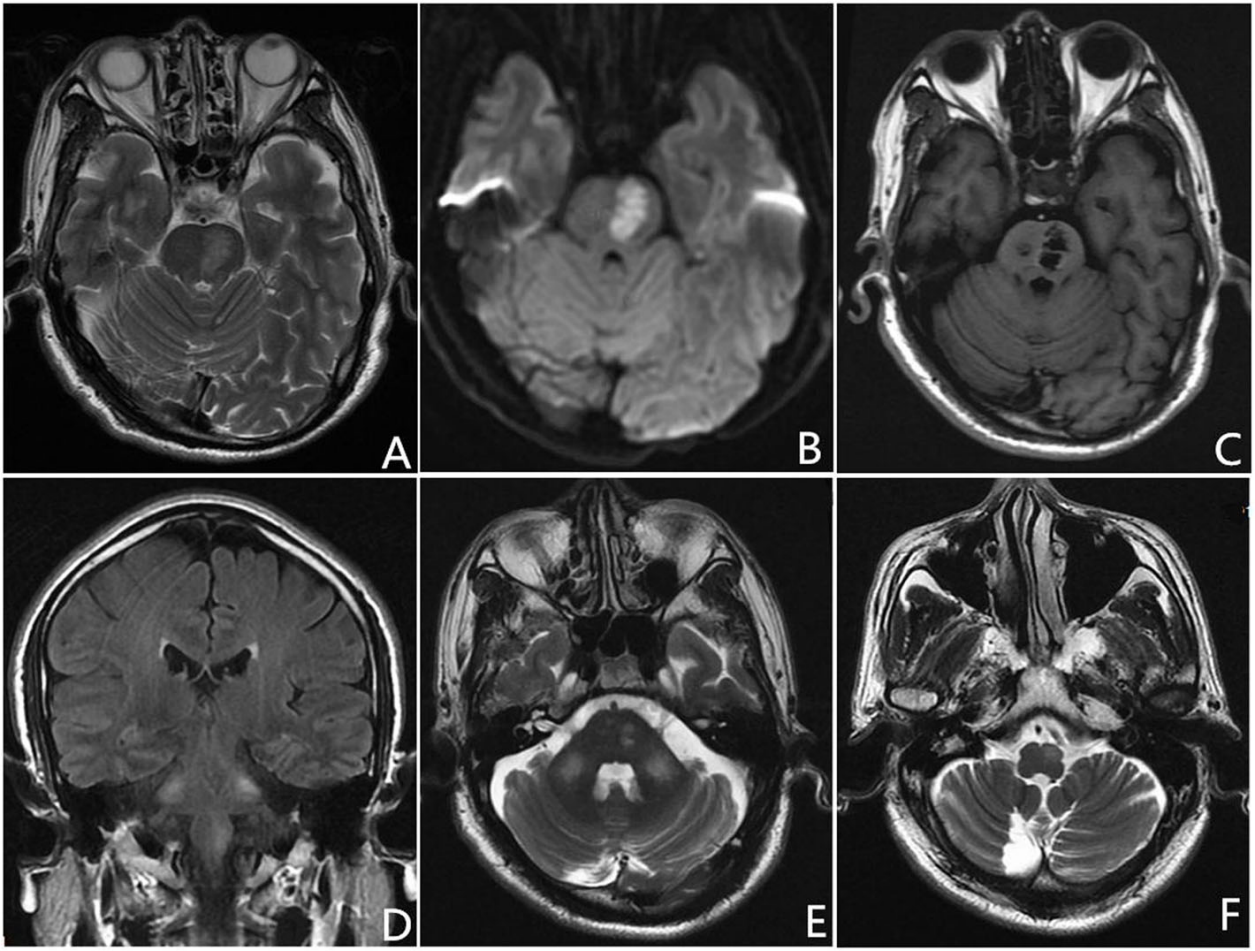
Axial T2-weighted and DWI images show acute pontine infarction (a, b). On 3-month follow-up MRI, axial T1-weighted image demonstrates an old paramedine pontine infarction (c). Coronal fluid-attenuated inversion recovery and axial T2-weighted images reveal hyperintensiy in the left inferior olivary nucleus (ION) and bilateral middle cerebellar peduncles (MCPs) (d-f)
